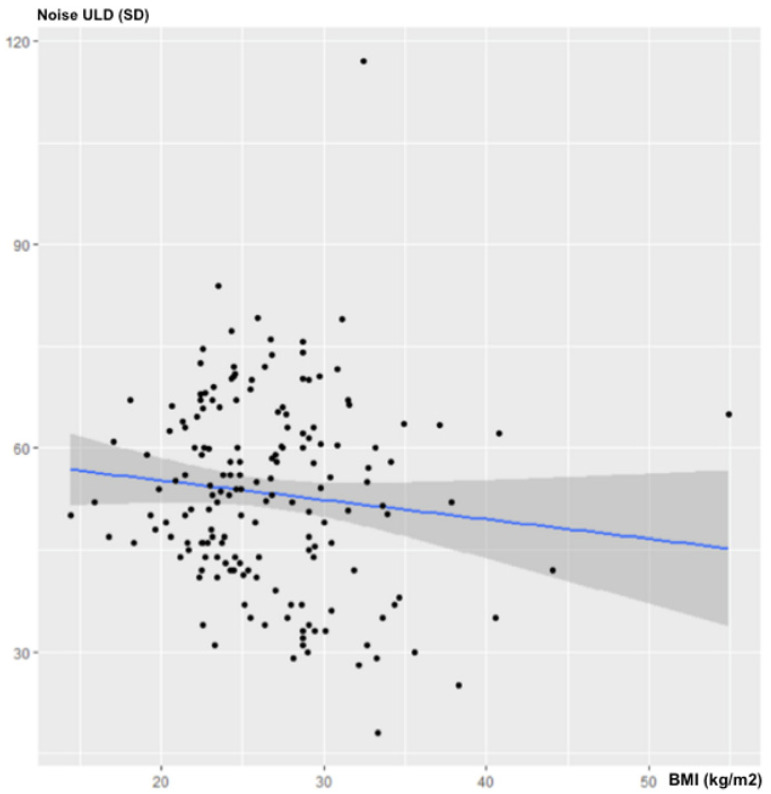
Influence of BMI on noise for ULD-CT.
